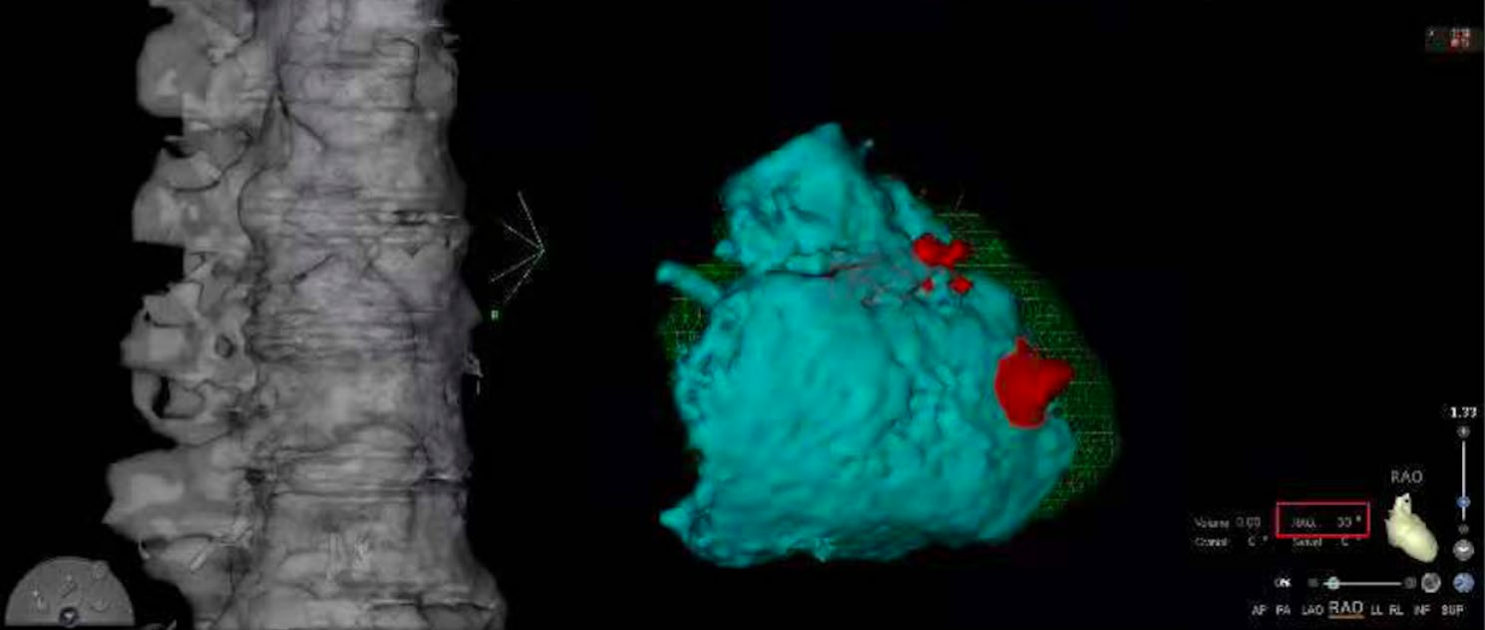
Cardiac CTA was performed 3 days after surgery and showed an endocardial defect in the region of the median septal sulcus of the right ventricle
